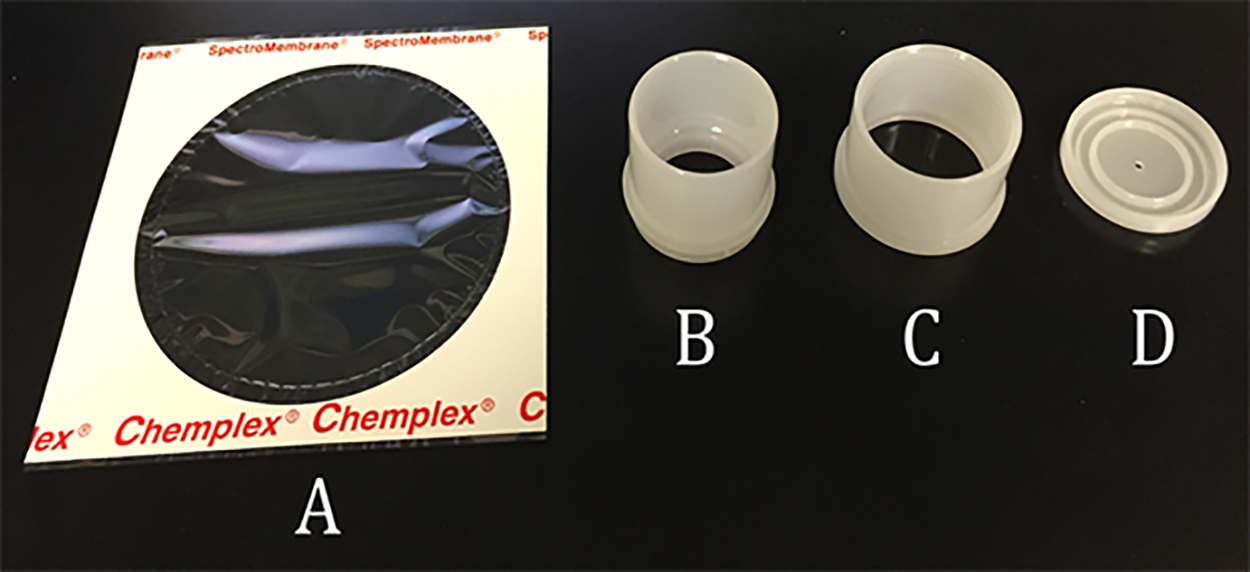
Mylar^®^ and individual cup components that make up the cell culture system. Mylar^®^ (A) provided a surface that allowed cells to grow in the same manner as if grown on standard tissue culture plastic, while also being thin enough to allow x-rays to pass through without significant attenuation. The Mylar^®^ was pressed between the inner (B) and outer (C) cup components with a ventilation cap (D) to allow proper air-flow.
